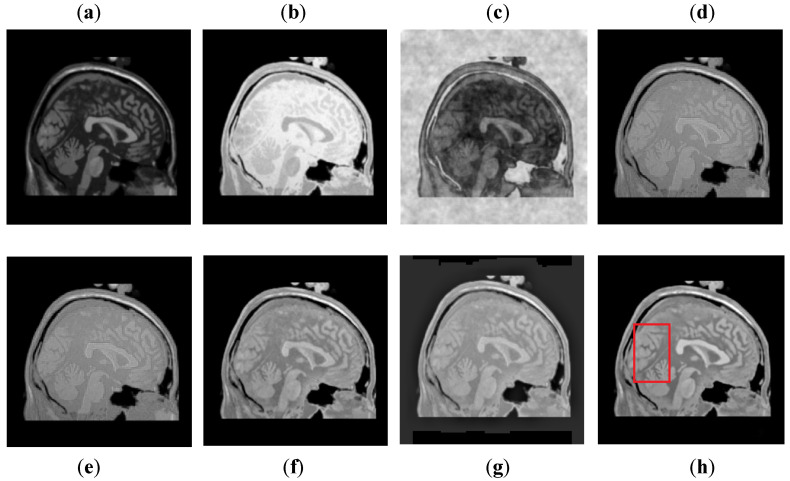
Data-5: (a) CT image, (b) MRI image, (c) DCT, (d) DWT, (e) NSST-SFT-PCNN, (f) CSR, (g) CNN, and (h) Proposed.
